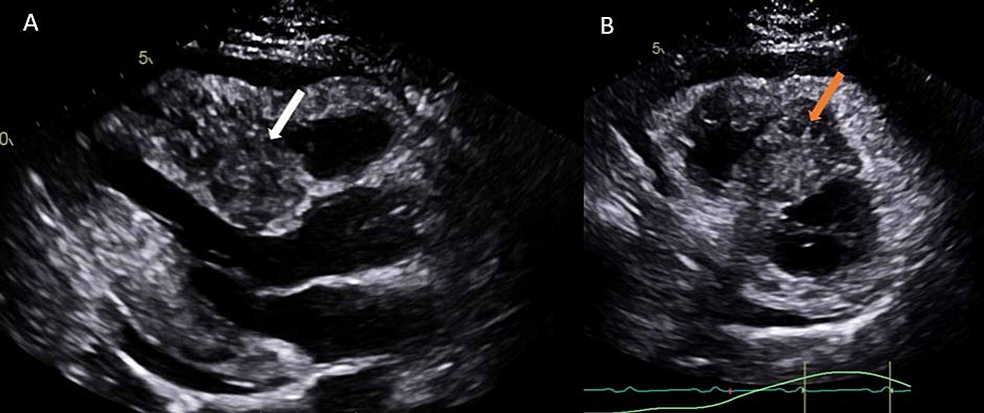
Two-dimensional TTE with parasternal long-axis view (A) showing hypoechoic mass (white arrow) in the interventricular septum and parasternal short-axis view (B) demonstrating spiculations (orange arrow) radiating out from the same central mass. TTE, transthoracic echocardiogram
